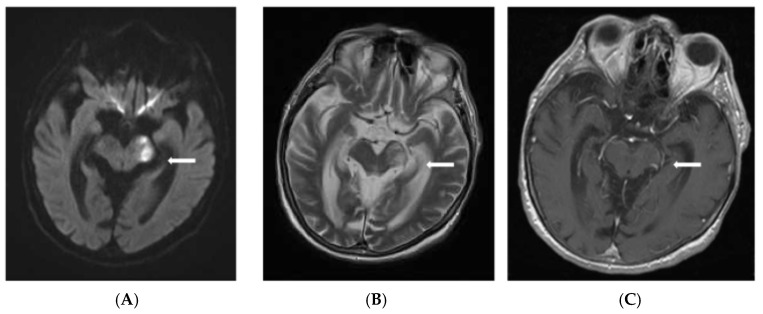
Lesional area located at the anterior portion (crus cerebri) of the left cerebral peduncle suggestive for acute ischemia, with water diffusion restriction (A) and high signal on T2-weighted MRI (B), hyposignal on T1-weighted Spin Echo MRI, without contrast enhancement with a slight increase in volume (C) marked with the white arrows.
